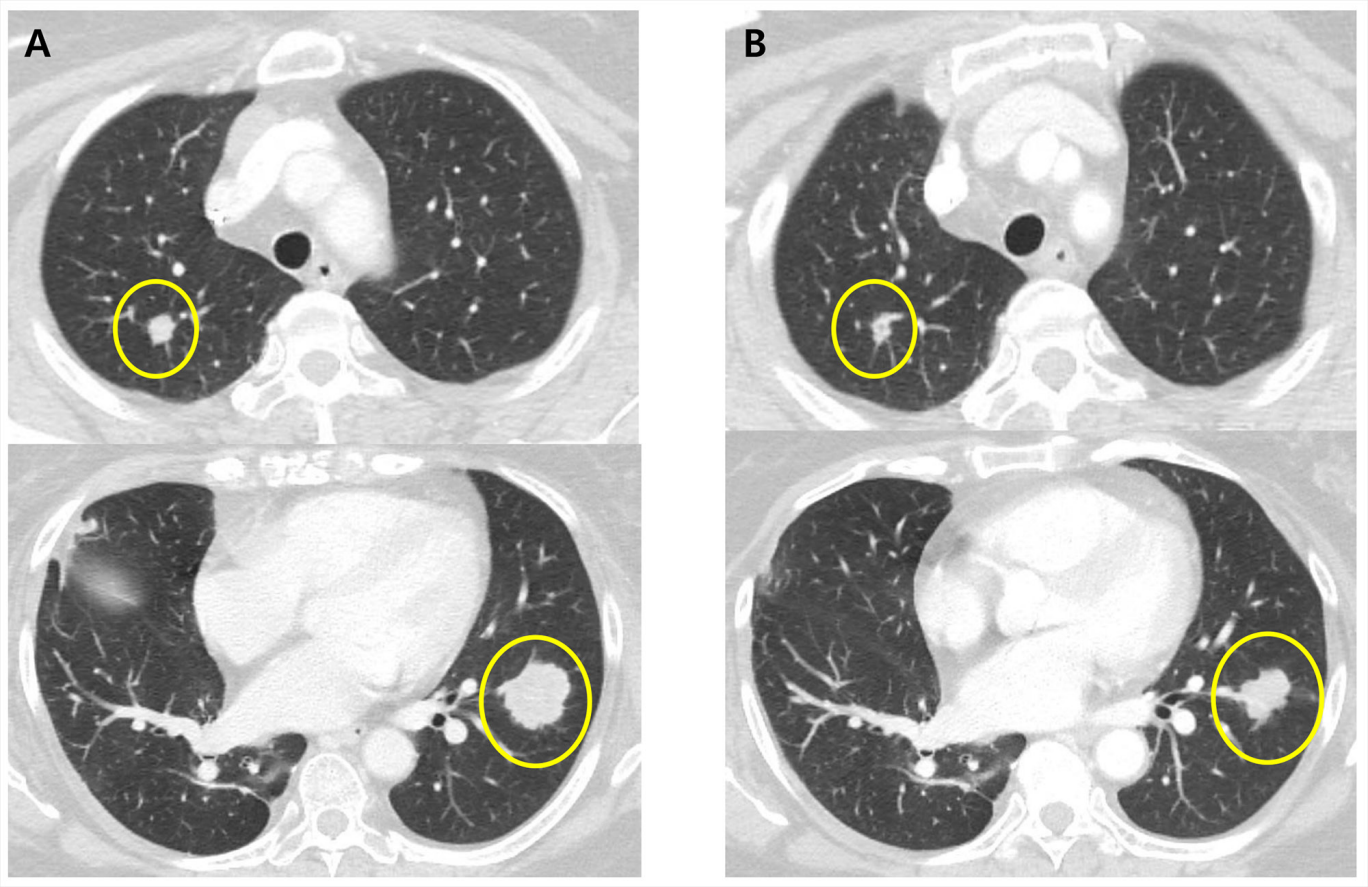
CT scans of the chest in patient with metastatic colorectal cancer harboring FLT3 amplification A. CT of the chest showing multiple metastatic nodules in the both lung before regorafenib B. CT of the chest showing response to treatment with regorafenib.
